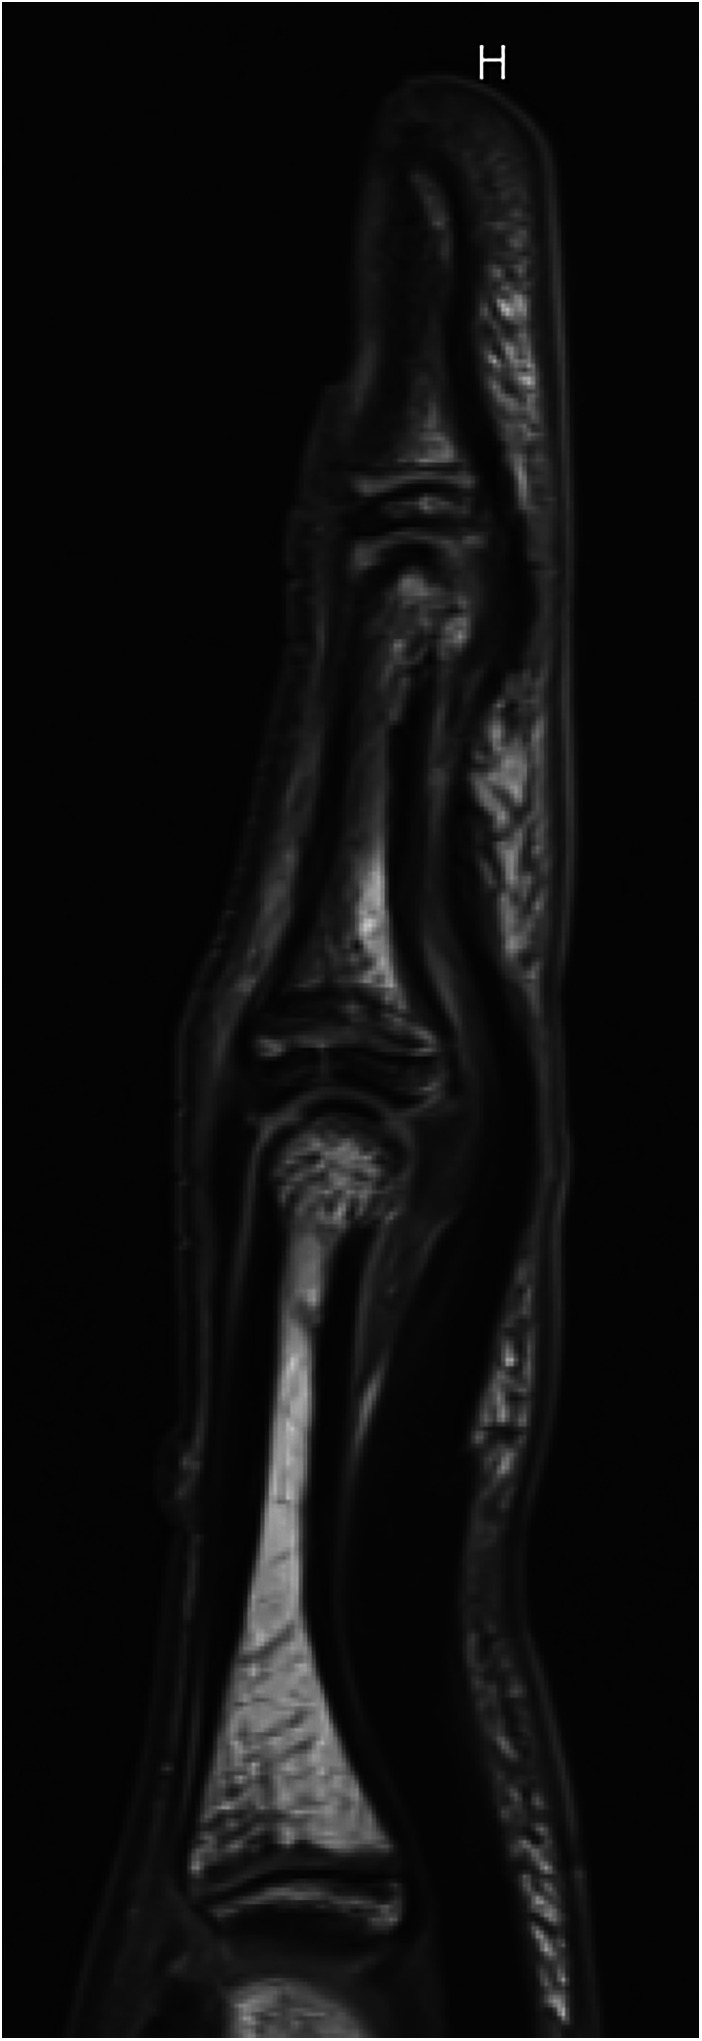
Radiographic sign 2. Non-displaced dorsal fracture of the epiphyseal-metaphyseal-complex (EPM) of the middle phalanx base (5, 9). (13 y old boy, left middle finger).
